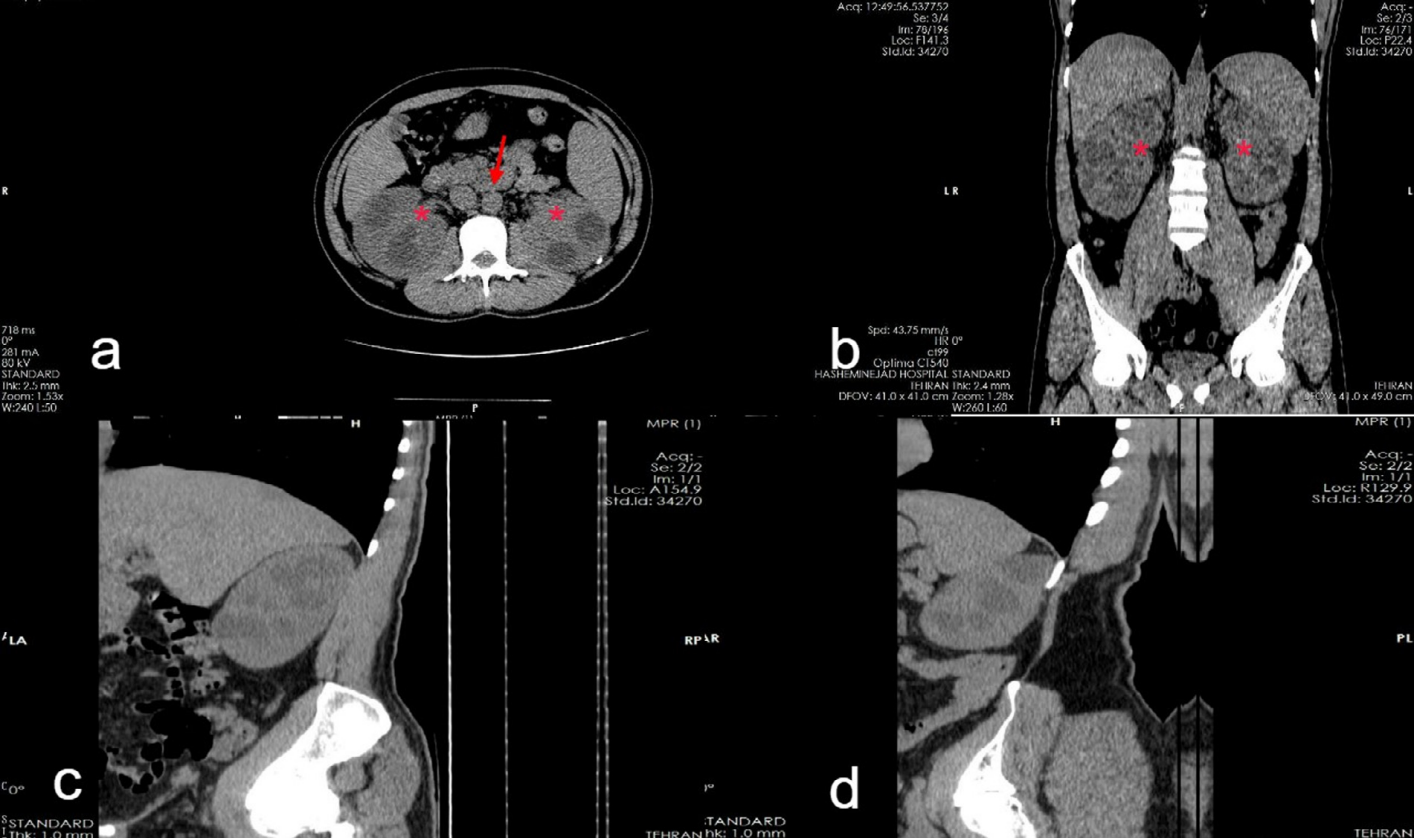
The nonenhanced CT scan shows diffuse severe hydro calyx in both kidneys with collapsed renal pelvises due to renal sinuses fibrotic tissue (*), also normal appearance retroperitoneum (arrow) is noted. (C) Curved sagittal view of right kidney with severe diffuse hydro calyx. (D) Curved sagittal view of left kidney with severe diffuse hydro calyx.
